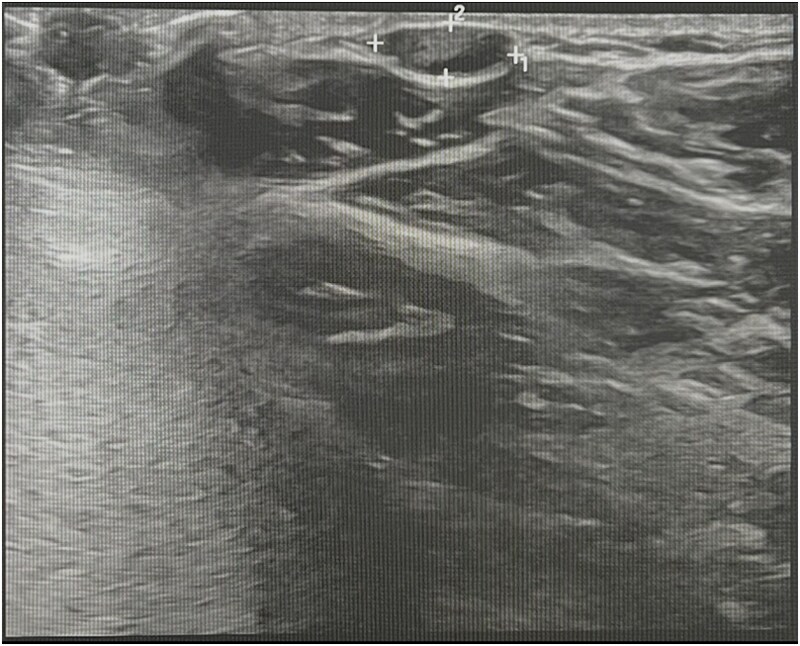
Ultrasound image showing the lesion.
